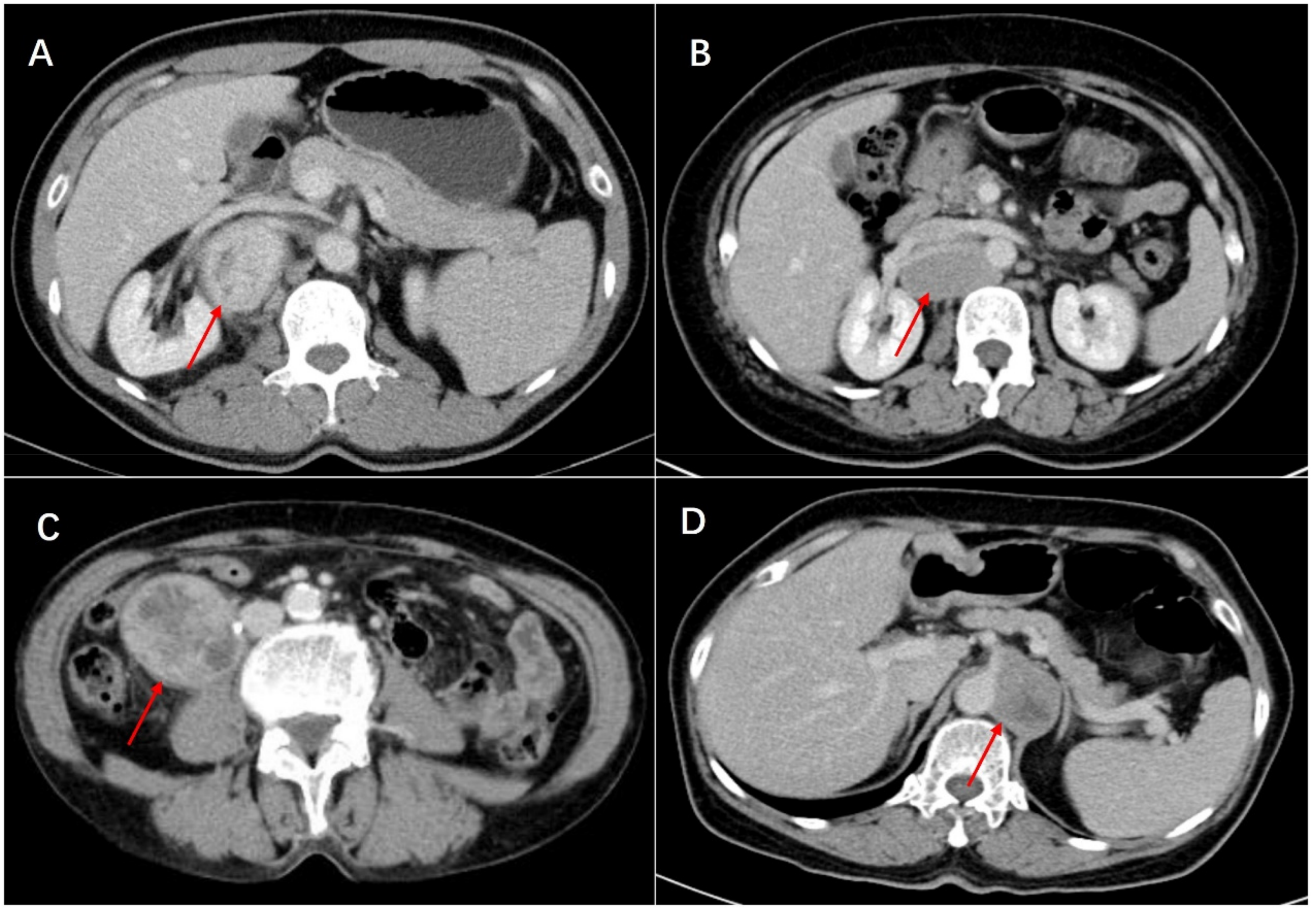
Venous phase of castleman disease (A), lymphoma (B), leiomyosarcoma (C) and paraganglioma (D) in enhanced CT scan. This lymphoma is highly similar to Castleman disease and surrounds the renal vessels, leading to a high risk of needle biopsy. Ultimately, surgical resection was performed, and postoperative pathology revealed invasive B-cell lymphoma.
